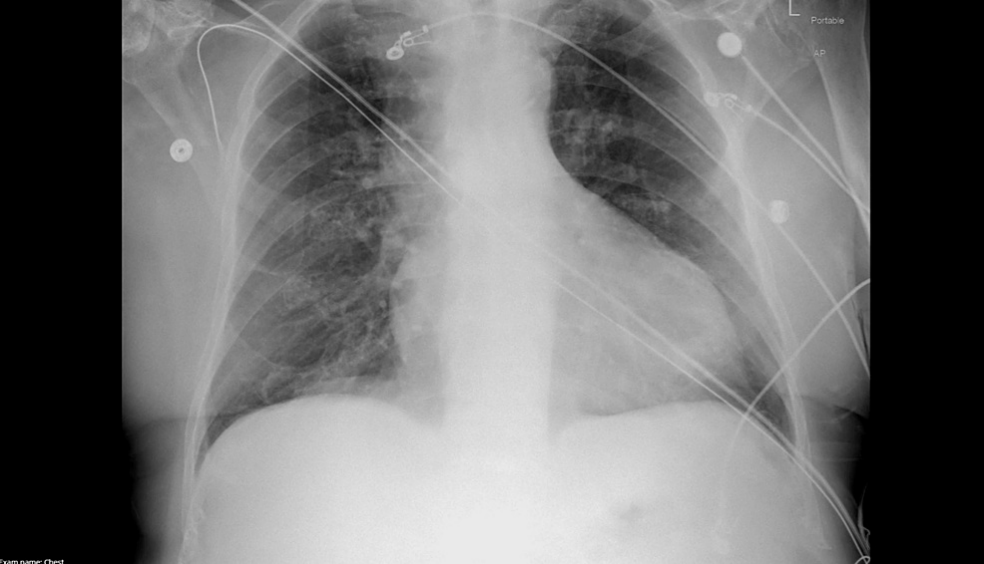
Initial chest X-ray significant for mild cardiomegaly with left ventricular prominence
